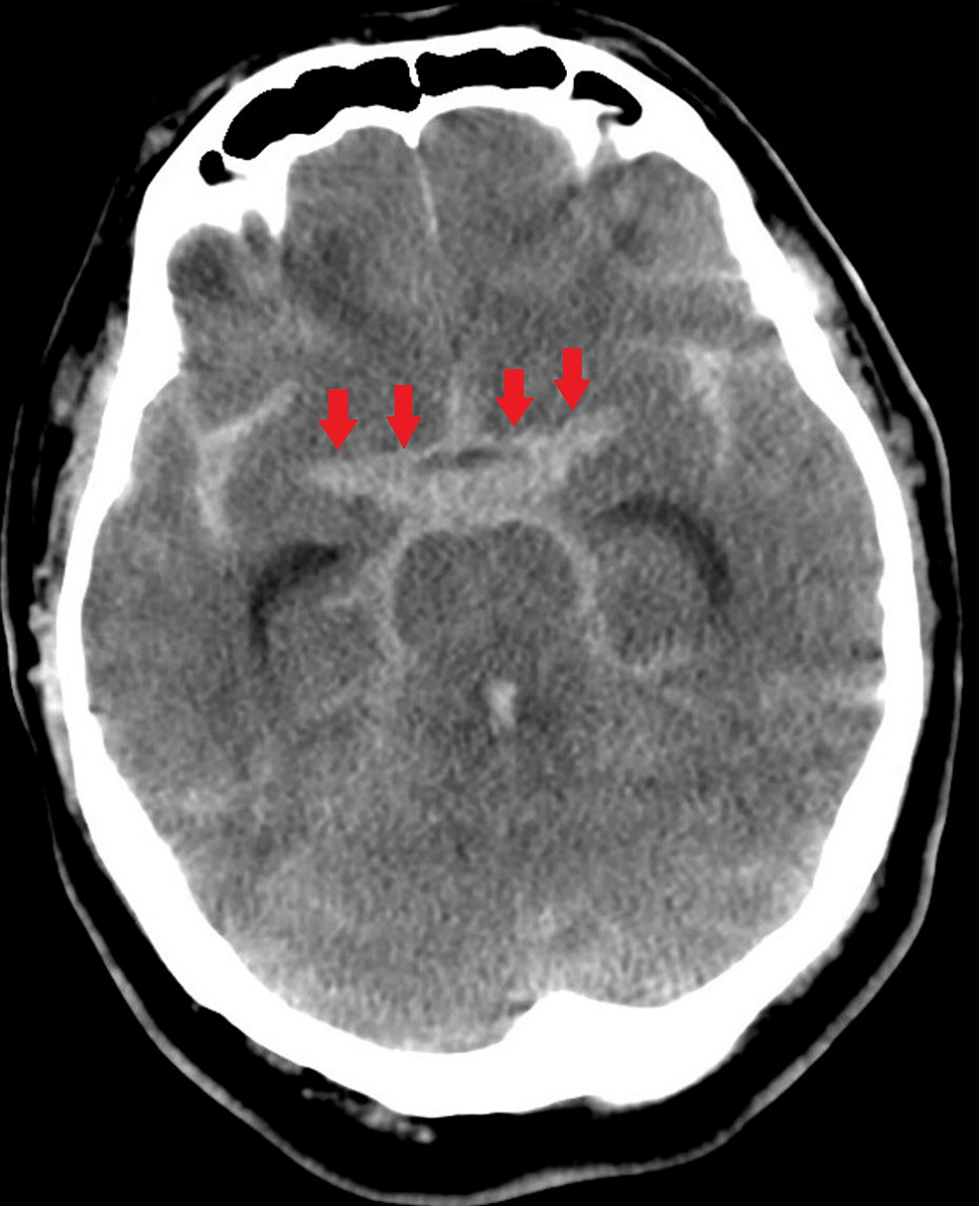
Head computed tomography Head computed tomography showed subarachnoid hemorrhage (Fisher’s classification 3)
